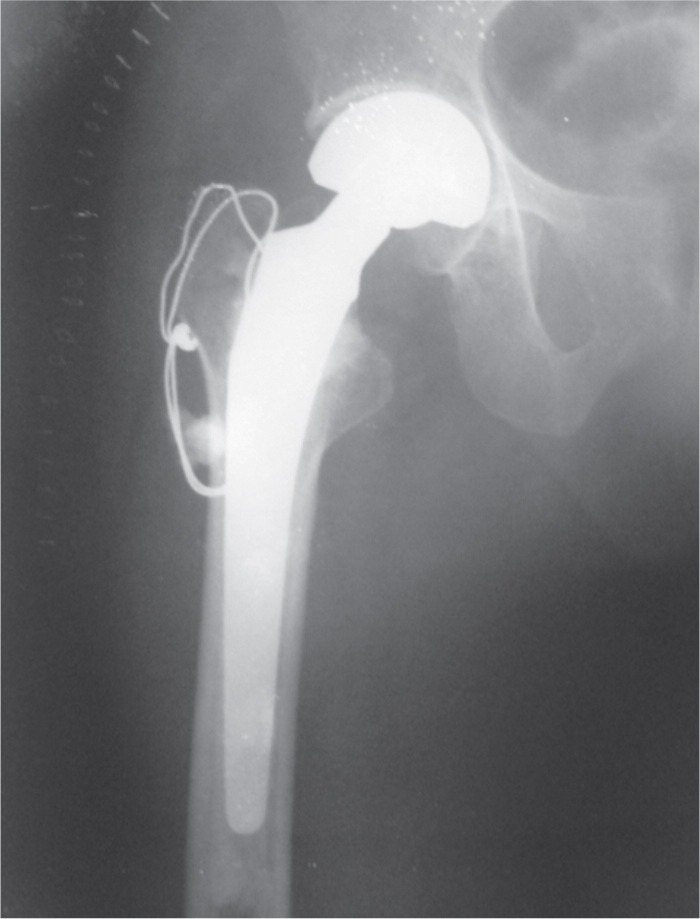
Postoperative Radiograph AP view.
